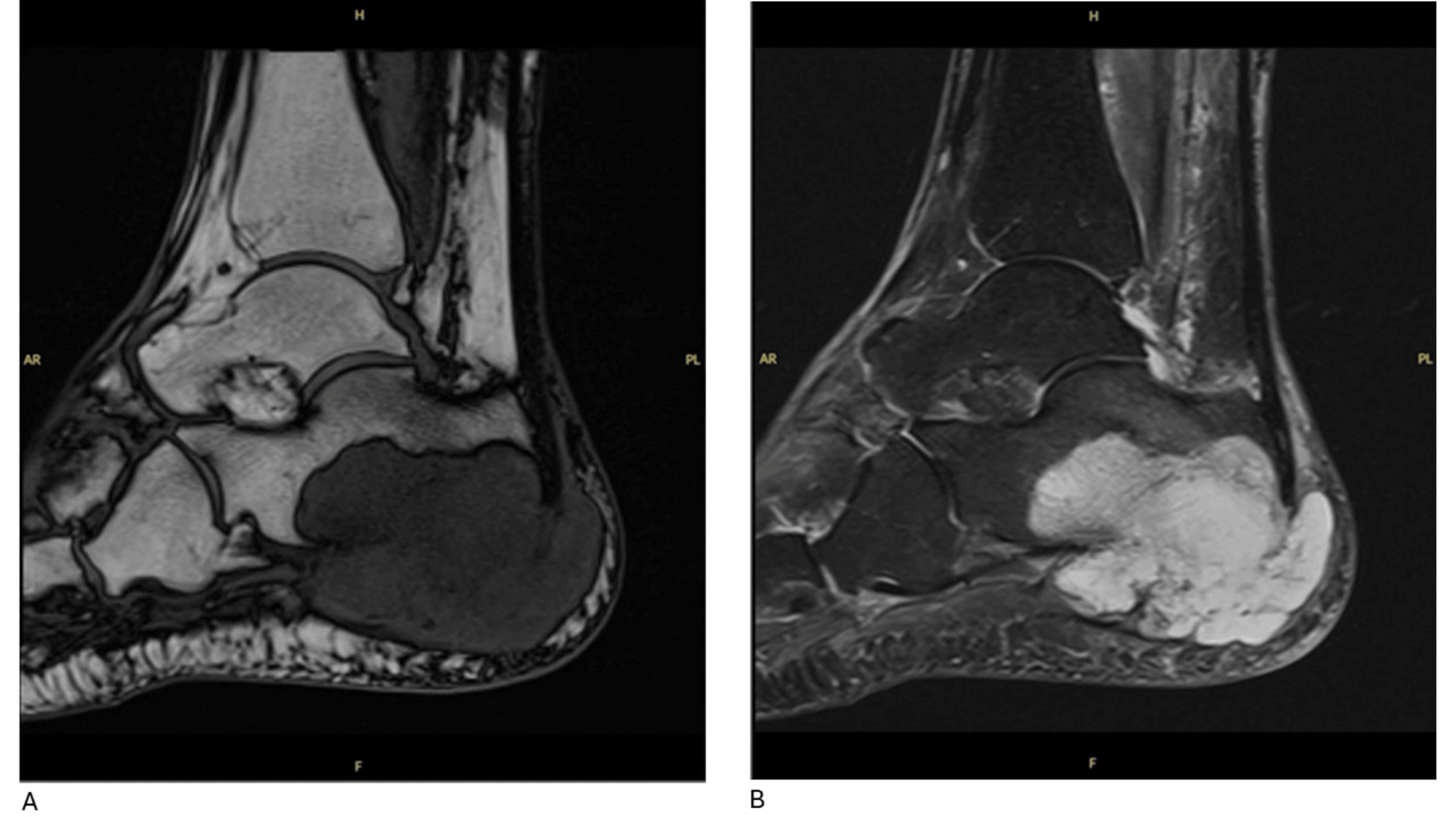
MRI of the patient’s right ankle showing a multiloculated cystic lesion (T1 and STIR sequences). (A) Magnetic Resonance Imaging (MRI) T1 sequence of the patient’s right ankle demonstrating a large multiloculated cystic lesion, (B) Magnetic Resonance Imaging (MRI) Short Tau Inversion Recovery (STIR) T1 sequence of the patient’s right ankle demonstrating the lesion with increased signal intensity.
